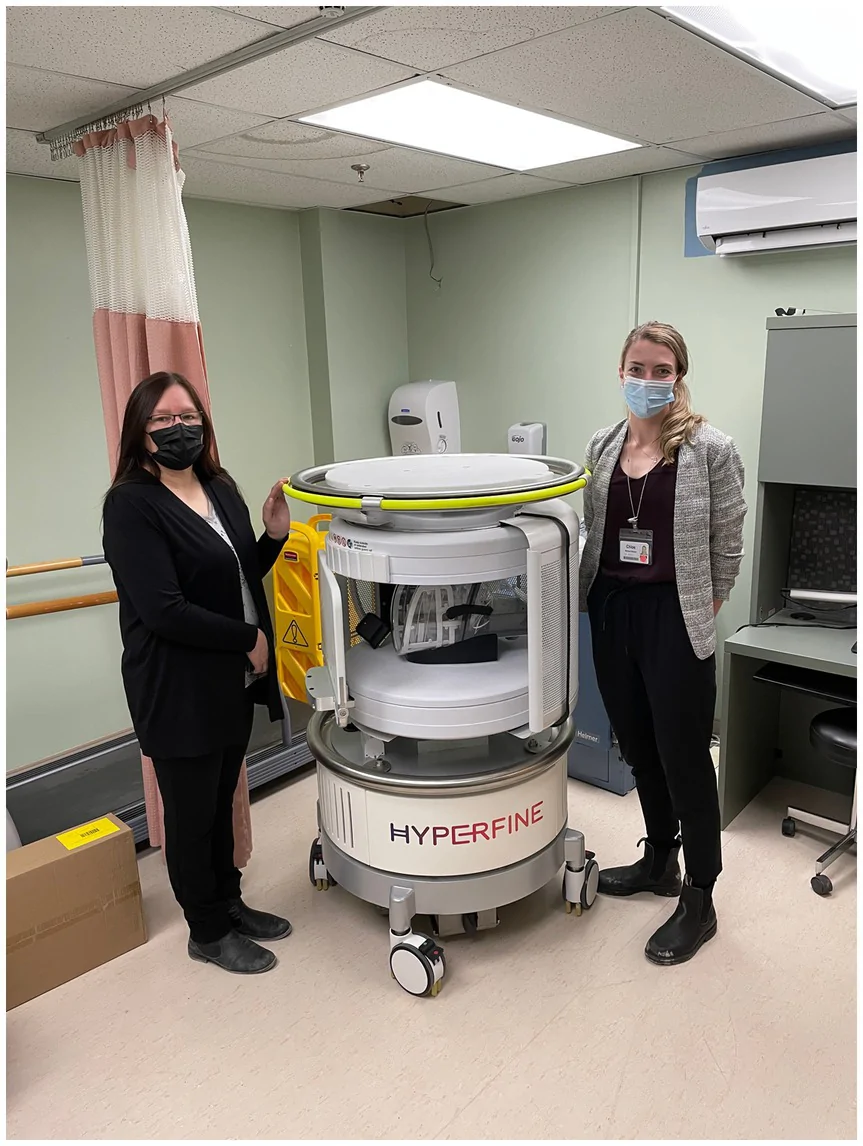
Portable MRI is an ultra-low-field bedside neuroimaging system that can be deployed in settings without conventional MRI infrastructure. The system can be housed in an existing clinical space, connected to standard power, and operated by trained local staff, with images transferred electronically for remote neuroradiology interpretation. Current pMRI systems are most useful for selected brain imaging indications, including screening or triage for larger intracranial abnormalities such as infarction, hemorrhage, hydrocephalus, mass effect, and midline shift. However, pMRI does not replace high-field MRI for all indications because of a lower signal-to-noise ratio, reduced spatial resolution, limited sequence availability, and the lack of routine contrast-enhanced, angiographic, perfusion, functional, or metabolic imaging. Portable MRI machine in Moose Factory with Dr. Elaine Innes (Chief of Staff, Weeneebayko Area Health Authority) and Chloe DesRoche (Queen’s University School of Medicine).
